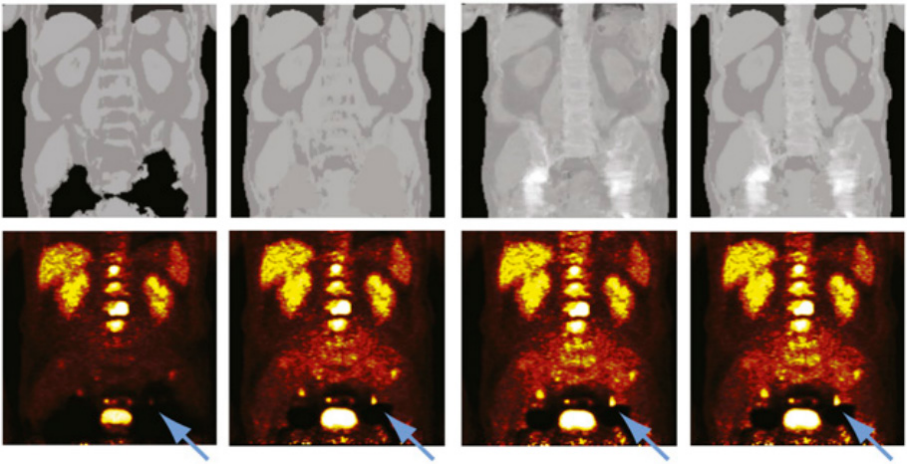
Attenuation maps (top) and PET images (bottom) for sample patient with hip prostheses. The used MRAC methods, from left to right, were SEG1, SEG2, AT&PR, and SEG2wBONE. Metal artifacts are due to bilateral hip replacement. Blue arrows denote lesion affected by presence of adjacent metal artifact when no correction was performed. This research was originally published in JNM. Bezrukov et al. “ MRI-Based Attenuation Correction Methods for Improved PET Quantification in Lesions Within Bone and Susceptibility Artifact Regions” *J Nucl Med*. 2013;54:1–7 ^©^SNMMI.^79^
